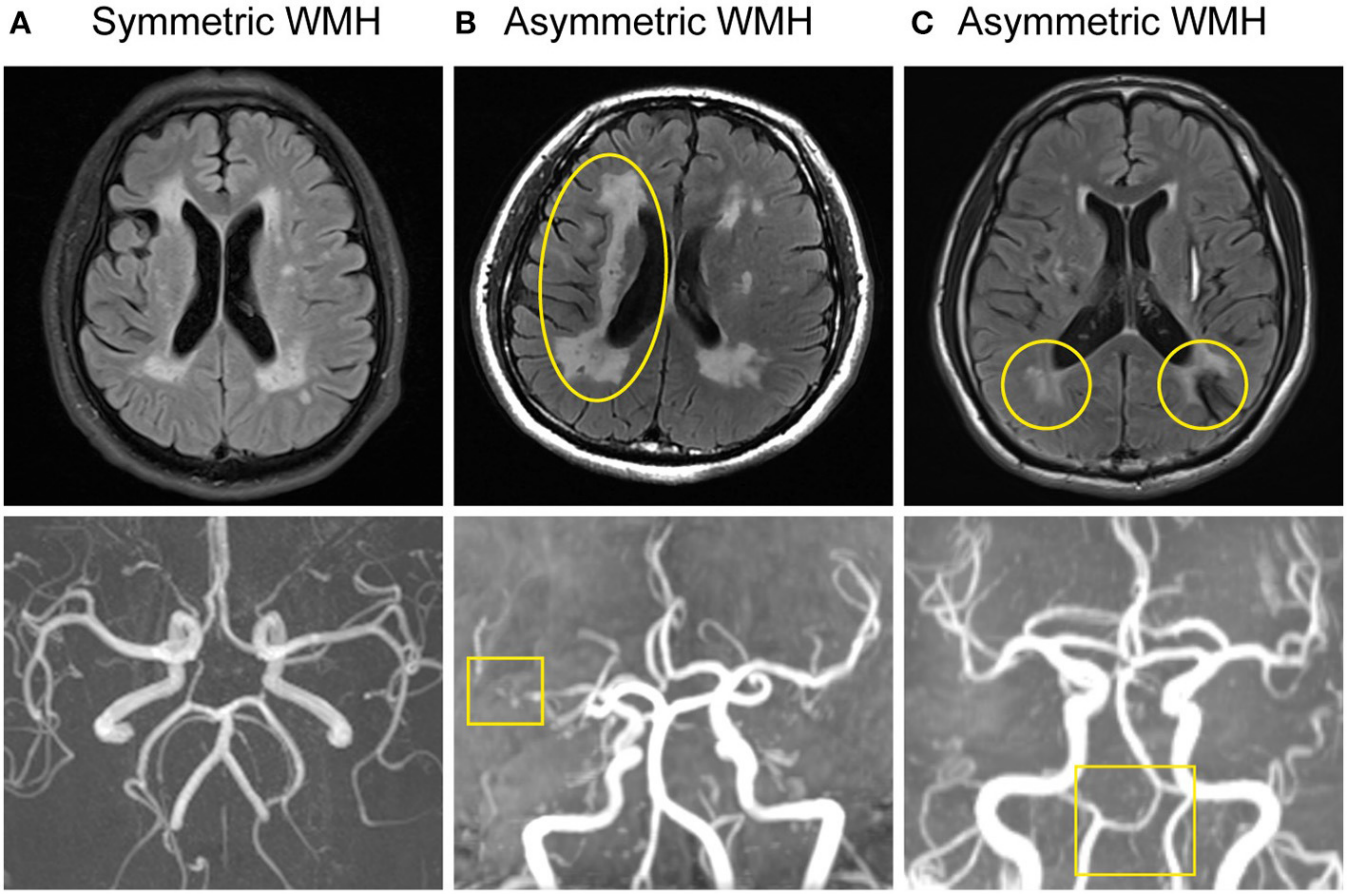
Large artery disorders and WMH distribution of CADASIL. (A) Normal intracranial large artery and symmetric WMH distribution. (B) MRA showed right MCA horizontal segment severe stenosis. Asymmetric WMH was seen on FLAIR, predominantly located in the right side, presenting with unilateral dominant WMH pattern (left-right asymmetric pattern). (C) MRA demonstrated VA hypoplasia. FLAIR displayed asymmetric WMH distribution, mainly in the posterior horn of the bilateral ventricle, presenting with a posterior WMH pattern (anterior-posterior asymmetric pattern).
